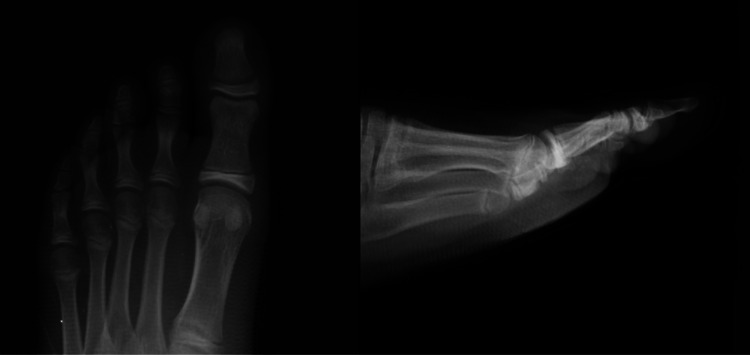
AP (left) and lateral (right) radiographs of the left foot in a 13-year-old male after stubbing his great toe, which demonstrates a Salter-Harris I fracture of the distal phalanx with dorsal displacement, plantar angulation of about 10°, and widening of the dorsal aspect of the physis. AP, anteroposterior.
